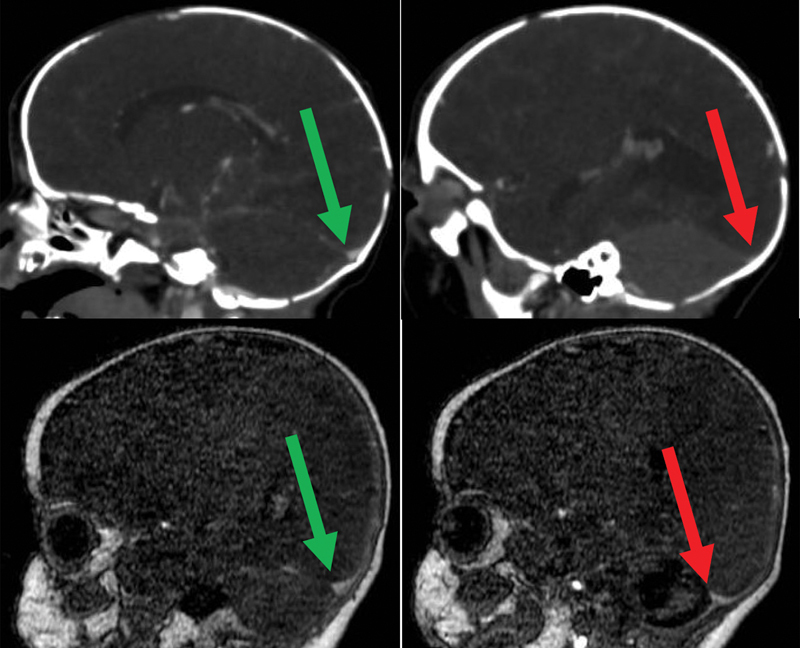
First row shows the computed tomography angiography at diagnosis: the green arrow indicates patent right transverse sinus and the red arrow indicates nonopacified proximal left transverse sinus. The second row shows the contrast-enhanced magnetic resonance angiography at follow-up: the green arrow shows the right patent transverse sinus and the red arrow shows the left patent transverse sinus.
